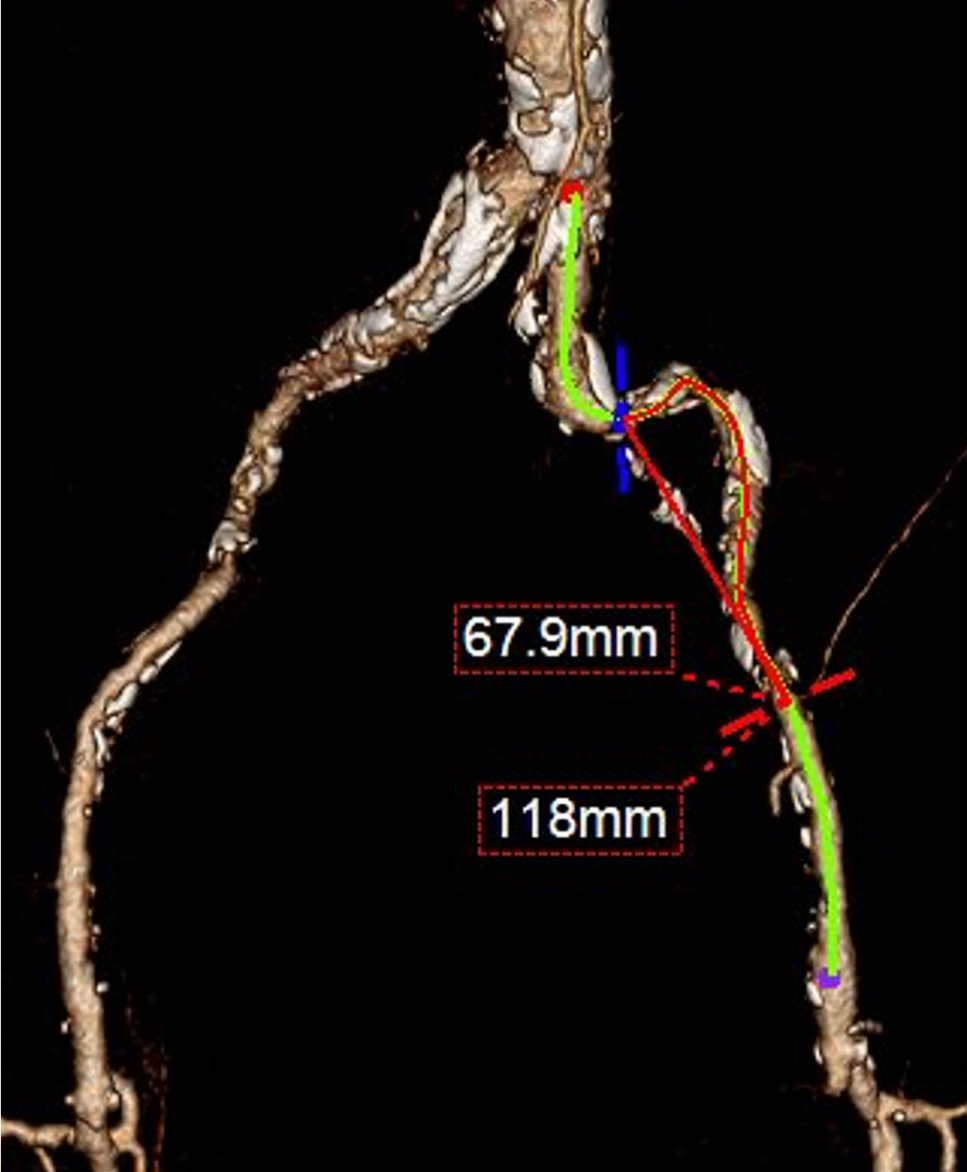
Three-dimensional CTA reconstruction of a left EIA disease. The tortuosity index is measured as the ratio between the centerline and the straight-line distance from the EIA origin to the circumflex arteries
